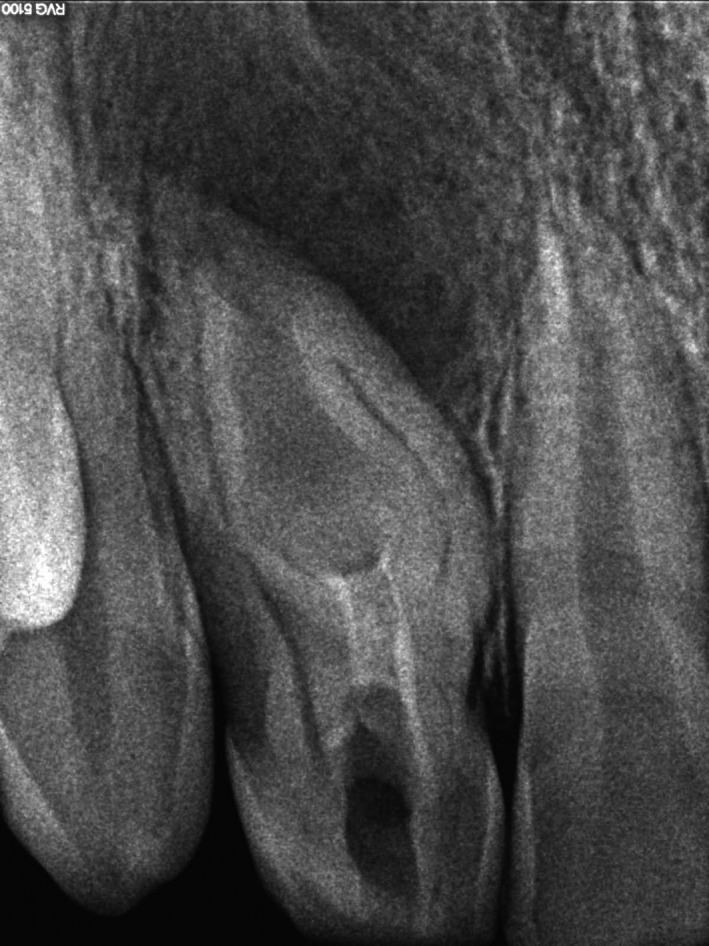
Preoperative radiography of the tooth #12. Note a huge radiolucent lesion in the periapical region and the invagination extending from the crown to the root apex
